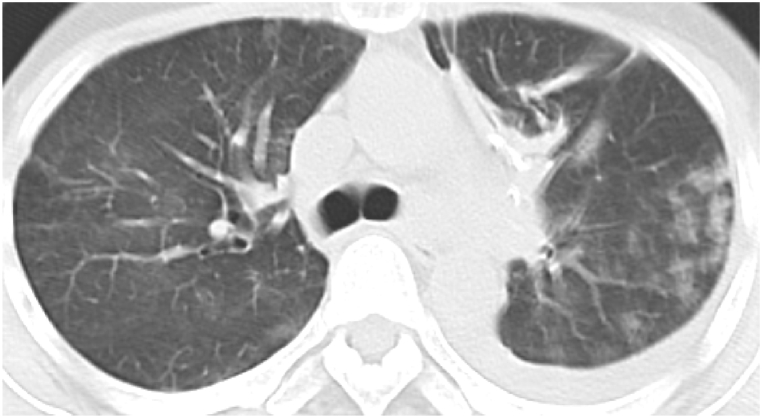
Chest CT during the previous hospital admission.
